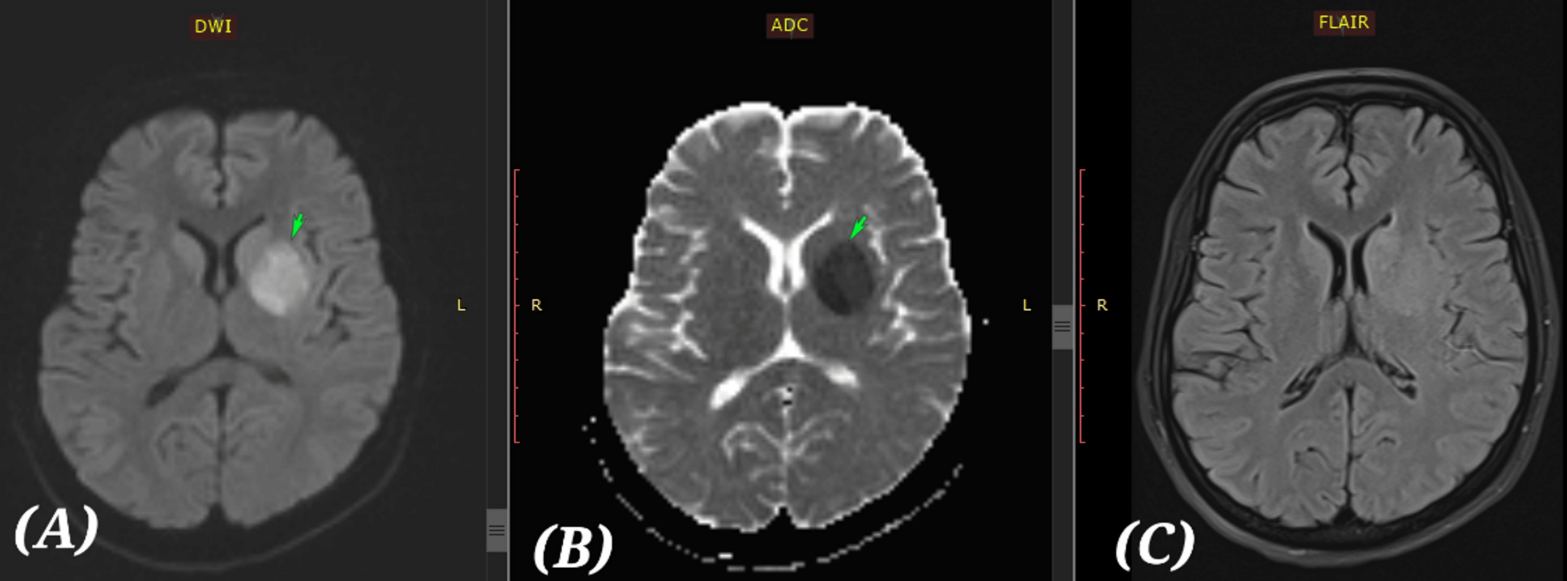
MRI brain-stroke protocol. Acute left MCA territory infarct, largest at the ganglio-capsular corona radiata region. (A) Diffusion-weighted imaging (DWI) showing a bright hyperintense signal (arrow marked) in the left basal ganglia/internal capsule region consistent with restricted diffusion suggestive of acute infarct. (B) Antibody-drug conjugate (ADC) with a corresponding dark hypo-intense area on the ADC map at the same site confirms true restricted diffusion suggestive of acute ischemic infarct. (C) Fluid-attenuated inversion recovery (FLAIR): There is no obvious bright signal seen in the same area, which means the infarct is very early (hyperacute, <4.5 hrs), before FLAIR changes appear.
